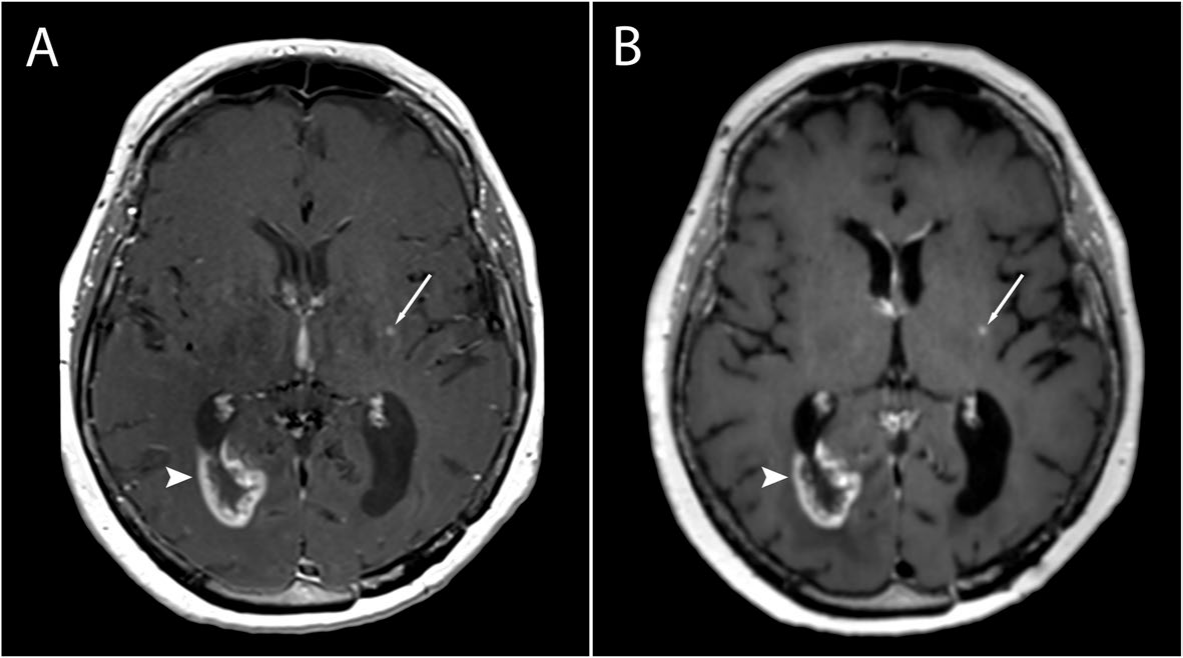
A T1-weighted SE sequence. B T1-weighted SPACE sequence. Metastases in the left basal ganglia (arrow) and right occipital region (arrowhead) in a 64-year-old female at 3 T, Gadovist 0.1 mL/kg. The SPACE sequence was acquired first
